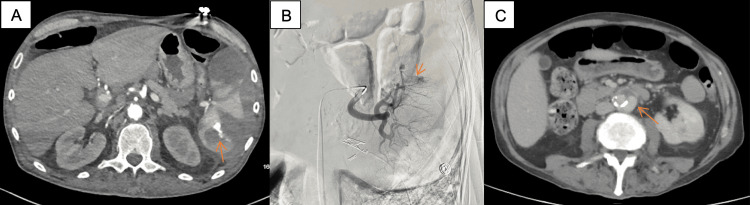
CT angiography chest abdomen and pelvis (A) Splenic artery pseudoaneurysm; (B) splenic artery angiography with third-order embolization of two inferior pole branches supplying a pseudoaneurysm and an area of active extravasation; (C) CT abdomen and pelvis with IV contrast showing abdominal aortic mycotic aneurysm.
